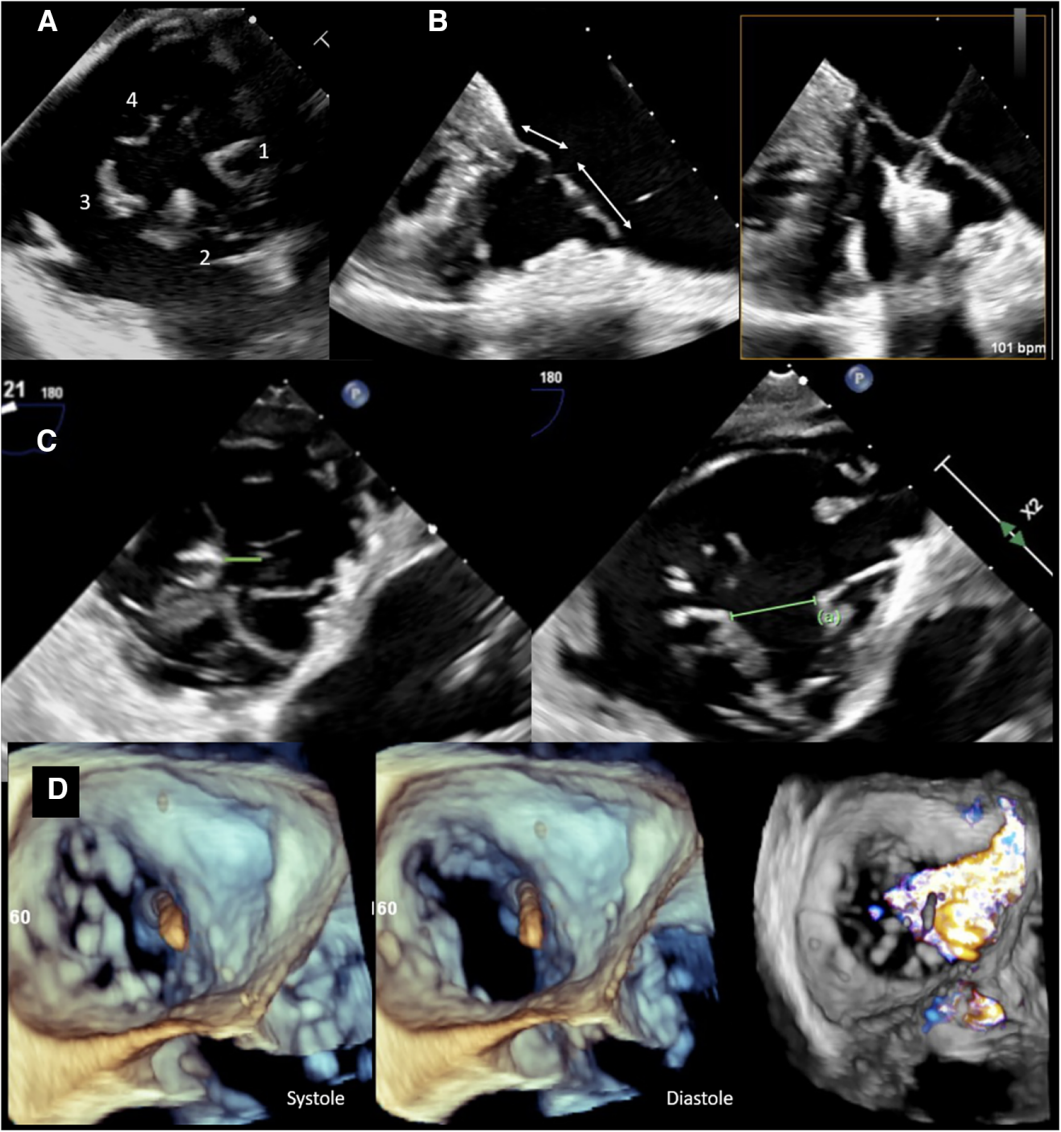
Preprocedural imaging of tricuspid valve. (A) Transesophageal view of a quadricuspid tricuspid valve with a (1) septal, (2) anterior, and (3,4) two posterior leaflets. (B) TEE view demonstrating septal and anterior tricuspid valve leaflets. There is sufficient length for TEER (>7 mm, left) on screening imaging, and during the procedure (right). (C) TEE images showing a reasonable coaptation gap (0.6 cm) on the left vs. a large coaptation gap (1.5 cm) between posterior and septal leaflets. The latter is an unfavorable feature for transcatheter edge-to-edge repair techniques. (D) 3D TEE image demonstrating an ICD across the TV impinging on the septal leaflet resulting in displacement of the leaflet and lack of coaptation in the systole. Color Doppler image on the right shows torrential TR with a wide base extending from anteroseptal to posteroseptal commissure.
